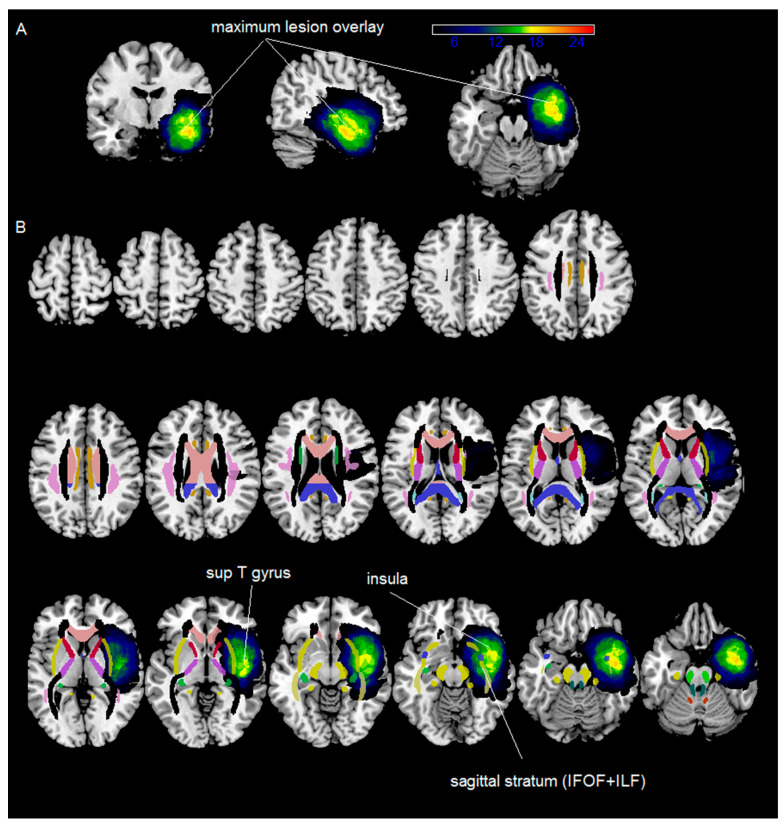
Brain areas that were maximally invaded by the tumor in the whole patient sample. (A) shows the maximum lesion overlay of all the patients’ volumes of interest drawn on their lesion volume. The color bar represents the number of cases, with yellow–orange indicating the maximum overlay. (B) shows the same maximum lesion overlay which is displayed on axial slices of a T1-weighted template, along with the Johns Hopkins University (JHU) Diffusion Tensor Imaging (DTI)-based white matter atlases [27]. The colors represent the different white matter pathways according to the atlas. Data are displayed in neurological conventions.
